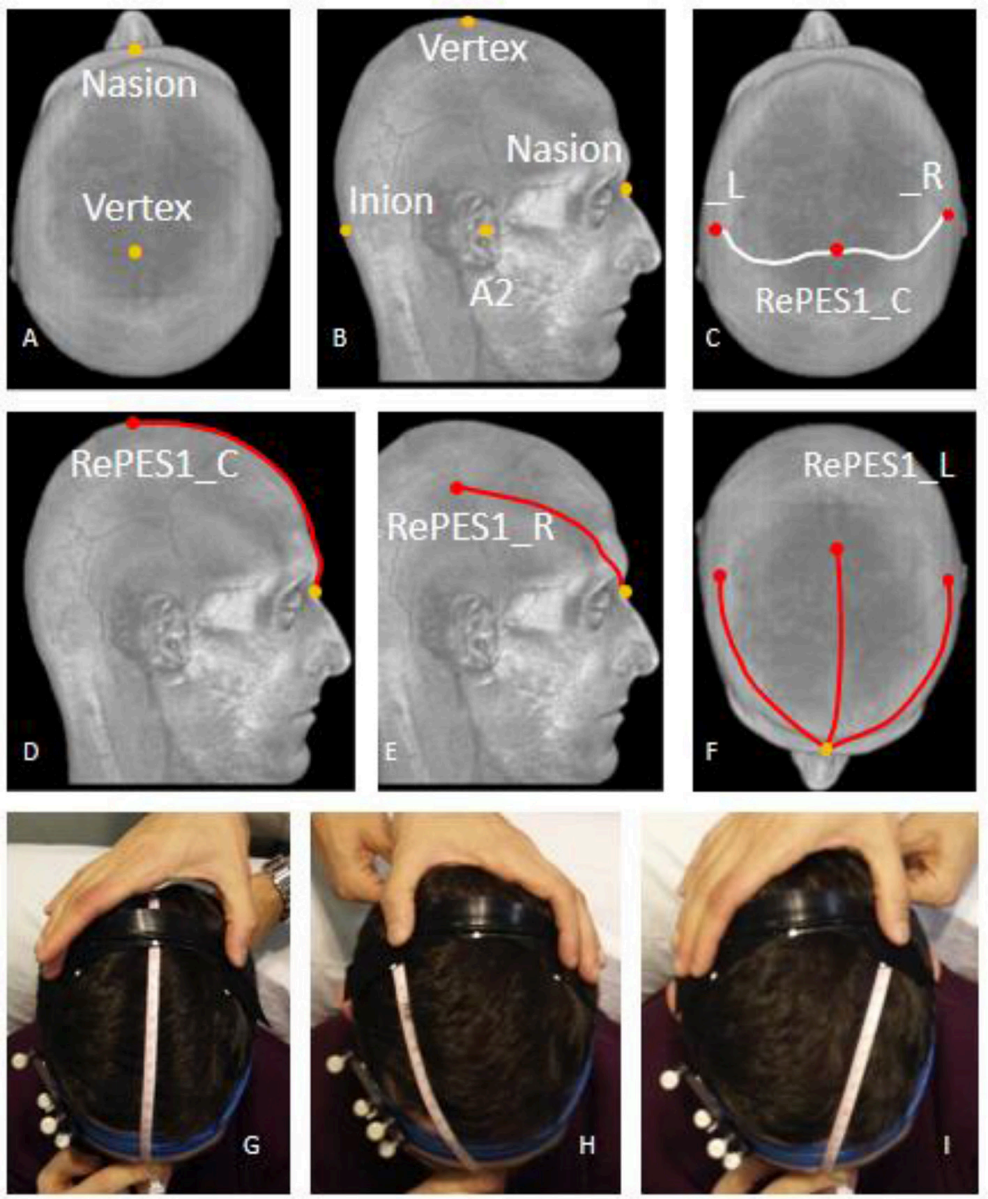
RePE positioning with 3D MRI-derived head space distances. As detailed in the Method section, representation of the curves on the scalp (A–F), whose lengths derived from the individual 3D head model allow positioning RePE (G–I).
